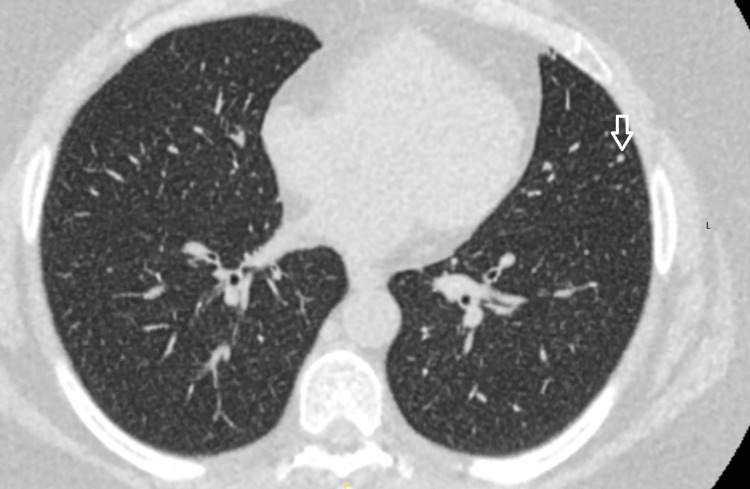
Low-dose computed tomography imaging showing a 3-mm nodule on the left side
